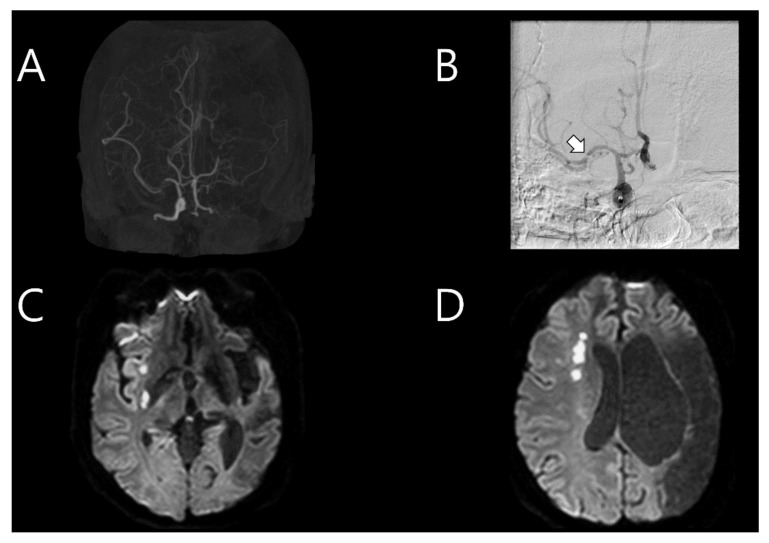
(A) Brain computed tomography angiography conducted on the second event which occurred after 5.5 years, showing recently formed severe stenosis in the M1 portion of the right middle cerebral artery (MCA), with left MCA occlusion present from the past stroke event. (B) Immediate cerebral angiography showing intramural hematoma and intimal flap in the M1 portion (arrow) of the right MCA. (C,D) Follow-up brain magnetic resonance imaging showing a multifocal acute infarction in the right MCA territory.
